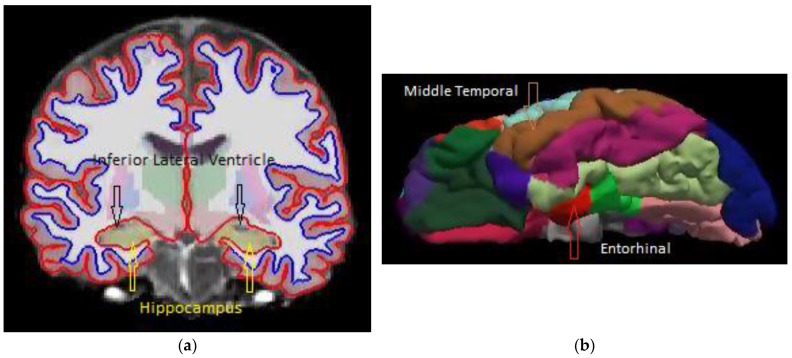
(a) A coronal slice of a young healthy subject’s T1-weighted MRI image showing the locations of the hippocampus and inferior lateral ventricles. (b) A surface map of the same young healthy subject’s cortical parcellation showing the entorhinal and middle temporal regions.
